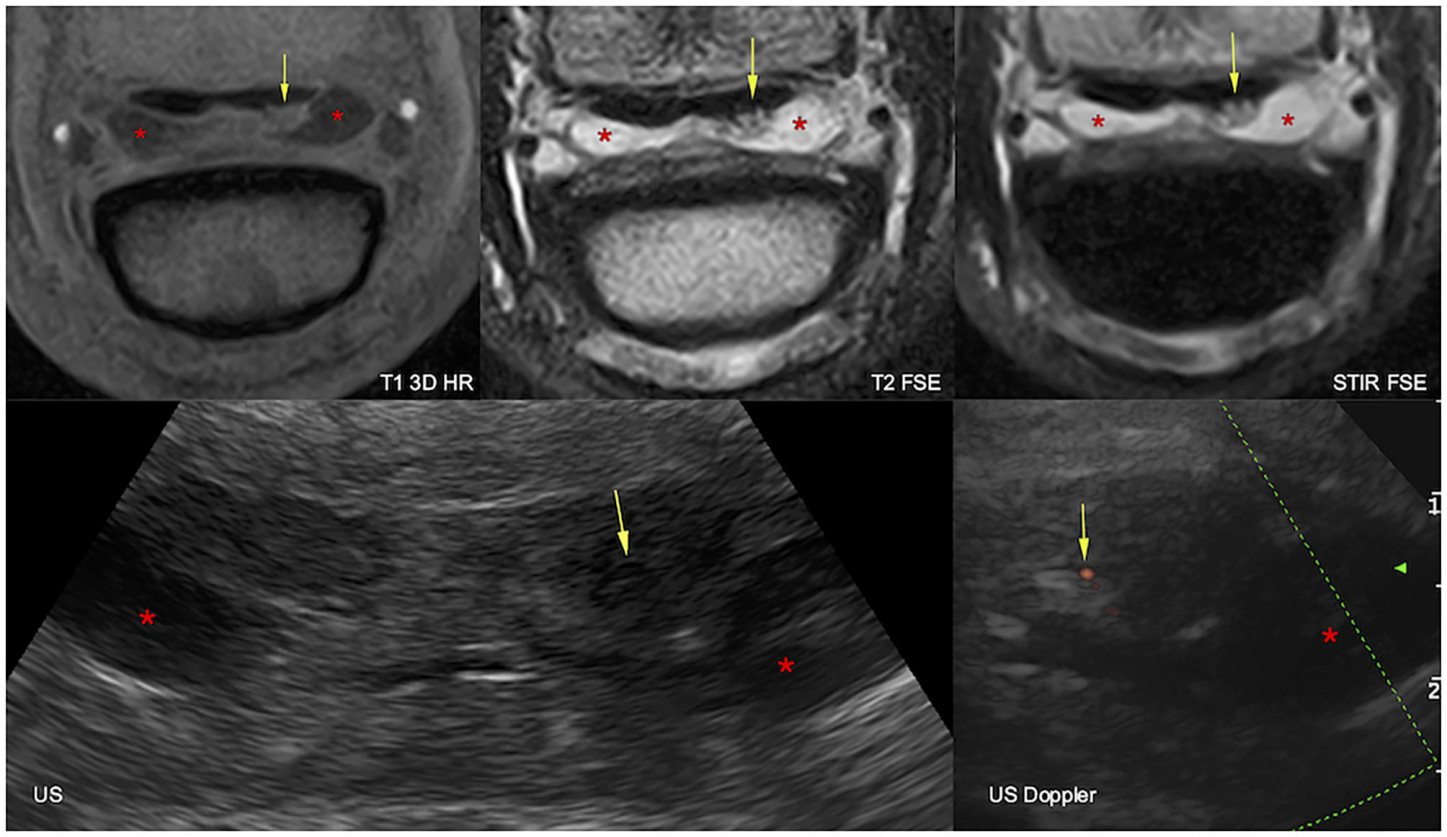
Transverse magnetic resonance imaging (MRI, top) and ultrasonographic (bottom) images of the right front foot at the suprasesamoidean level: lateral is to the right and dorsal to the bottom. The lateral lobe of the deep digital flexor tendon is increased in size and deformed by a dorsal lesion, hyperintense in all MRI sequences, hypoechoic, and vascularized at ultrasound (arrows). The navicular bursa is moderately effused (asterisks). T1 3D HR, high resolution tridimensional gradient echo T1 weighted sequence; T2 FSE, fast spin echo T2 weighted sequence; STIR FSE, fast spin echo short tau inversion recovery weighted sequence; US, ultrasonography; US Doppler, Doppler ultrasonography.
